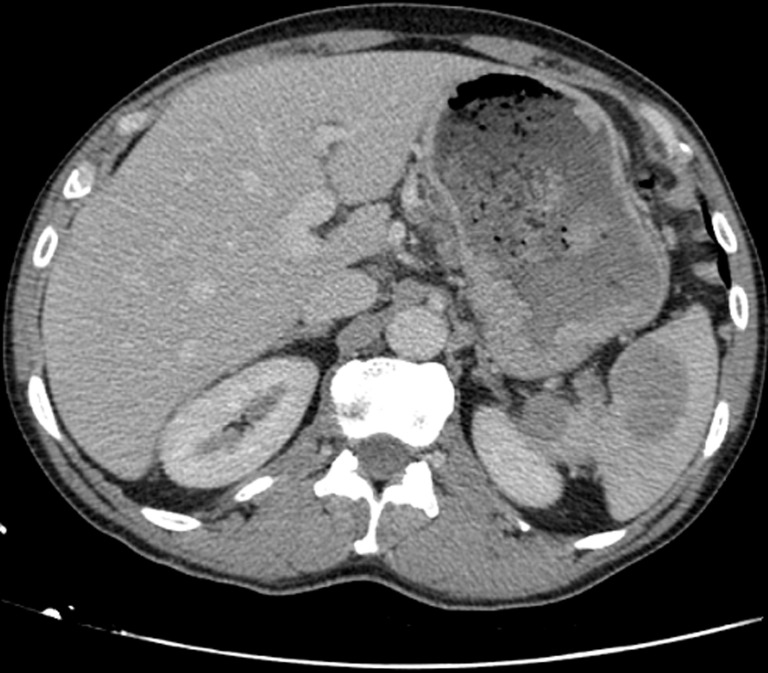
abdominal CT scan findings
